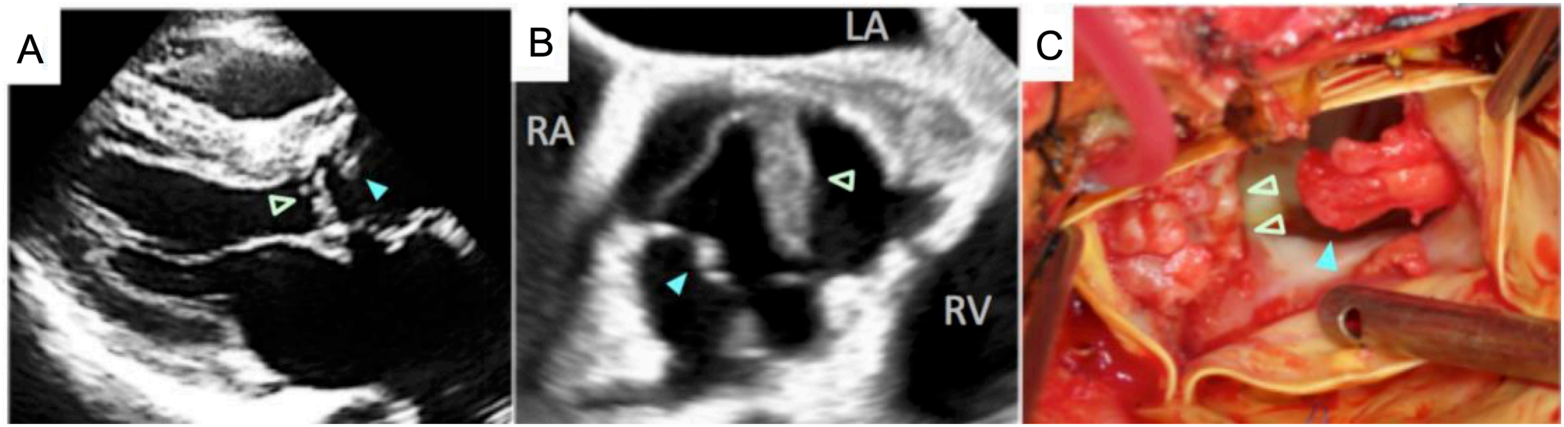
Aortic valve with vegetation (blue arrow: right coronary cusp; green arrows: left coronary cusps). A: Transthoracic echocardiography. B: Transesophageal echocardiography. C: Intraoperative findings. LA = left atrium; RA = right atrium; RV = right ventricle.
